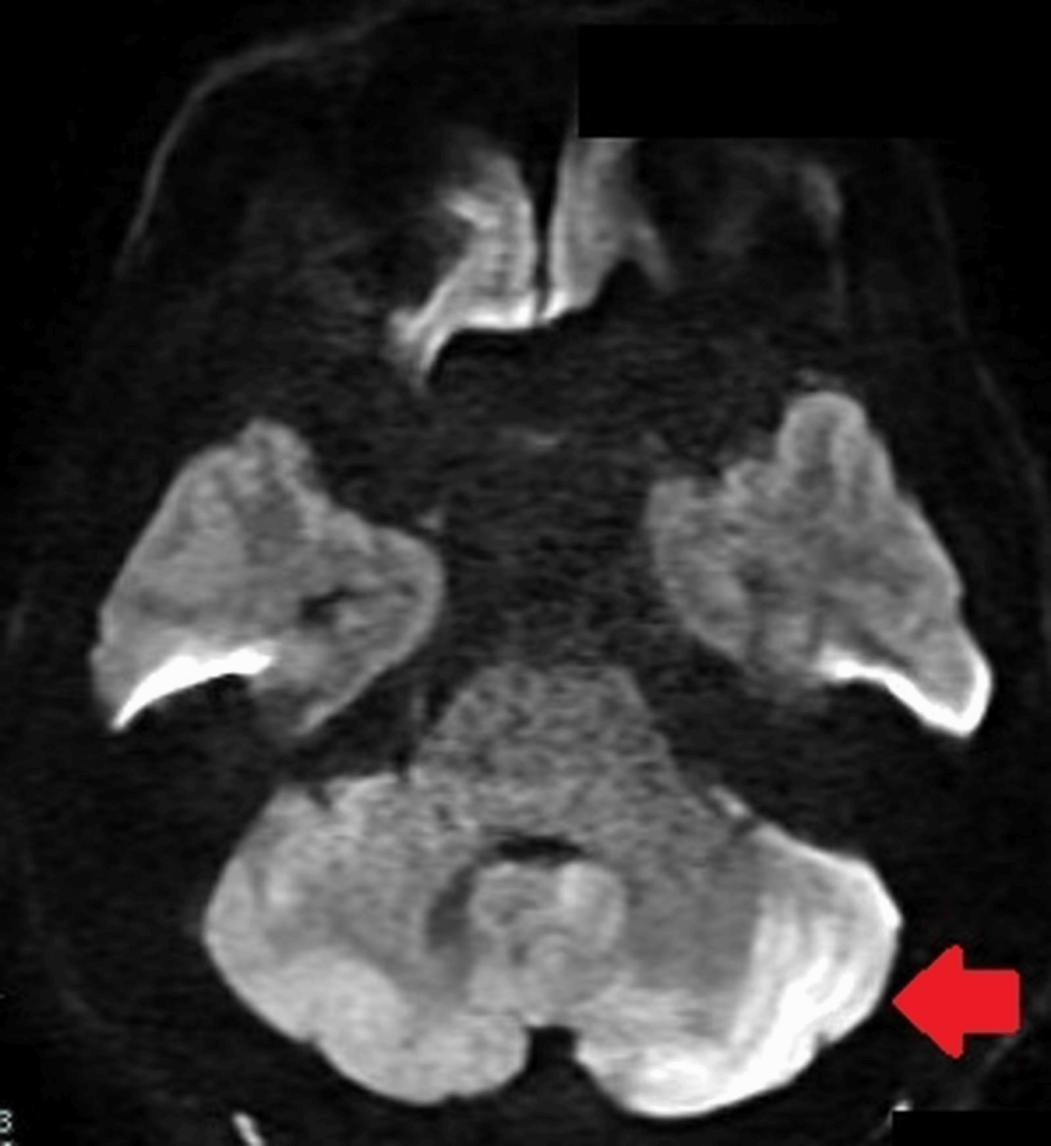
MRI DWI shows a hyperacute cerebral infarct in the posterior inferior cerebellar artery region (red arrow), suggestive of thrombus or dissection and hyperacute cerebral infarction. MRI: magnetic resonance imaging; DWI: diffusion-weighted imaging
